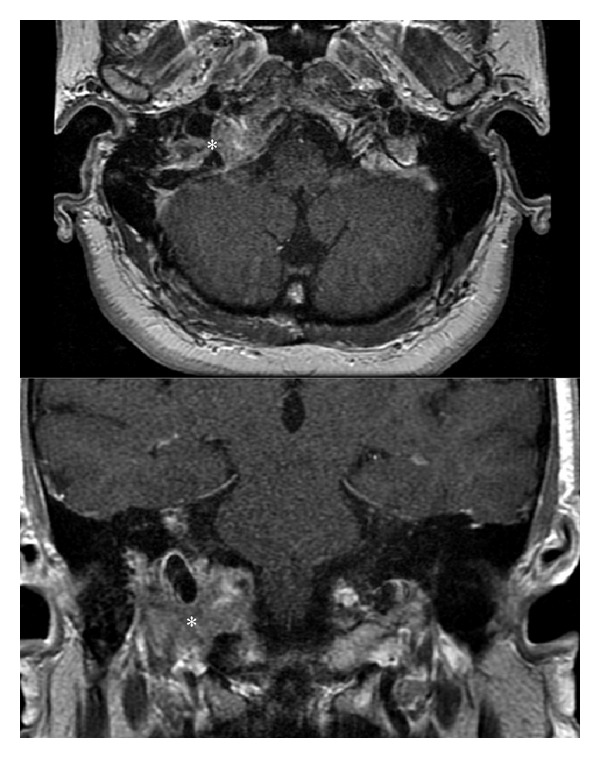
Axial and coronal contrast enhanced T1 MR images demonstrates a solid enhancing tumor at the right jugular foramina (∗) the lesion exhibits heterogeneous internal signal intensity given by the presence of internal flow-voids, resulting in the so-called “salt and pepper” appearance.
